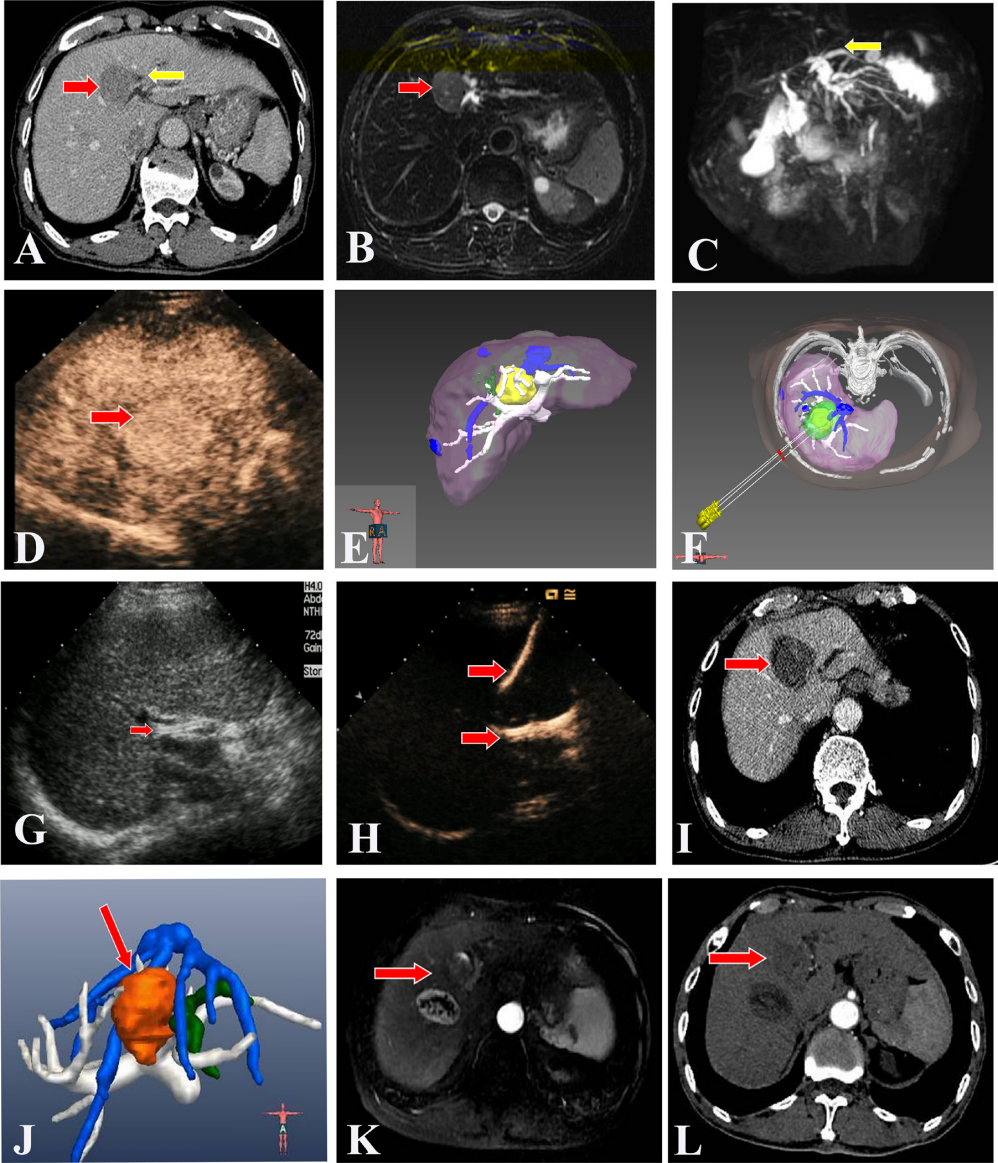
US-PMWA assisted by 3D visualization operation treatment planning and for a 74-year-old man with a larger HH-HCC (4.4 × 3.2 cm) (A, B, C) Pre-operation CT imaging showed a larger HH-HCC (red arrow). The left hepatic lobe intrahepatic biliary duct expansion (yellow arrow). Pre-operation magnetic resonance cholangiopancreatography (MRCP) imaging showed a larger HH-HCC (red arrow) and intrahepatic biliary duct expansion in the left hepatic lobe (yellow arrow). (D, E, F) Pre-operation CEUS imaging showed a larger HH-HCC with hyper-enhancement in artery phase (red arrow). Pre-operation 3D visualization treatment planning system showed the location of the tumor and the close relationship with the surrounding structures, and three antennas were need to ablate the tumor completely and safely. (Yellow: tumor; White: hepatic portal vein; Green: biliary duct; Blue: hepatic vein) (G, H, I) A PTCD tube was inserted percutanously into the left hepatic biliary duct before PMWA to prevent thermal injury of the duct, which was verified by CEUS examination. A significant ablation effect on the tumor was obtained by a series of PMWA, as shown on a contrast-enhanced CT image. (red arrow). (J, K, L) Post-operation 3D visualization treatment planning system evaluated the ablation effect with completely ablation without biliary duct injury. The contrast-enhanced MRI images 1 year after ablation showed complete tumor necrosis and the ablation zone shrunk gradually (red arrow). While there was a intrahepatic distant recurrence beside the primary tumor at 1 year after ablation and recieved another procedure. In Figure L, there was no local progress in the two ablation zones on contrast-enhanced CT images at 2 years after first ablation. (Brown: ablation zone; White: hepatic portal vein; Green: biliary duct; Blue: hepatic vein).
